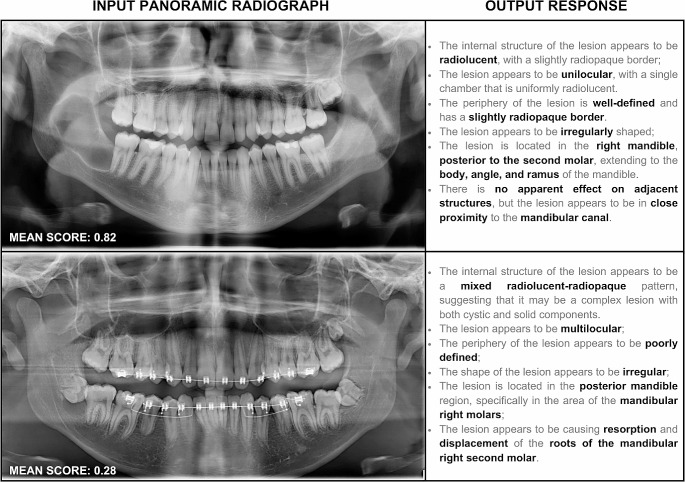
Representative panoramic radiographs and their corresponding divergent outputs from the GPT program, leading to a higher score (mostly correct output) in the top image and a lower score (mostly incorrect output) in the bottom image
